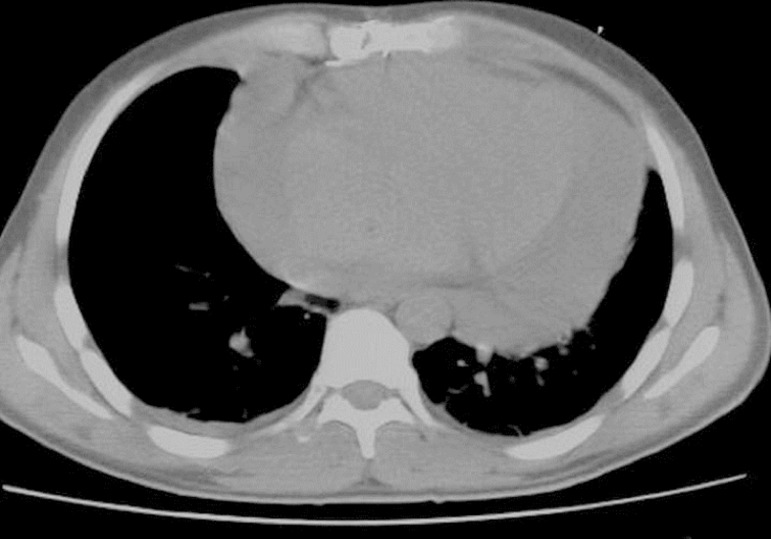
Marking the entry point with computerized tomography (CT).
